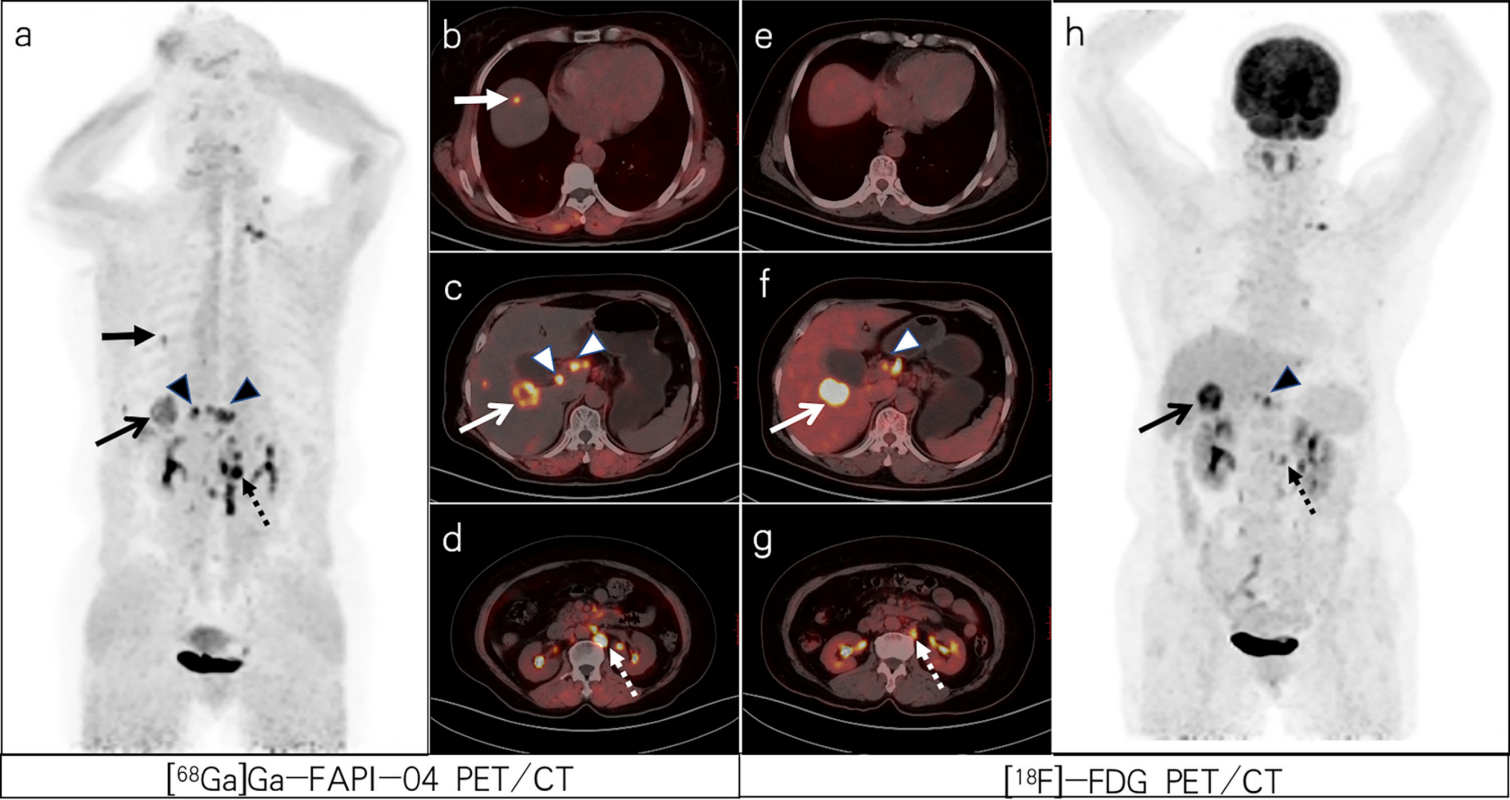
A 64-year-old woman with poorly differentiated gastric adenocarcinoma based on pathological biopsy under gastroscopy. Both [^68^Ga]Ga-FAPI-04 (A, C) and [^18^F]-FDG PET/CT (F, H) were negative in the primary focus. Compared with [^18^F]-FDG PET/CT (E–G), [^68^Ga]Ga-FAPI-04 PET/CT (B–D) resulted in a higher liver/background ratio and identified more liver lesions (slender arrows, SUVmax=6.3 vs. 11.3) and para-aortic lymph node metastases (dashed arrows, SUVmax=12.8 vs. 8.0).
